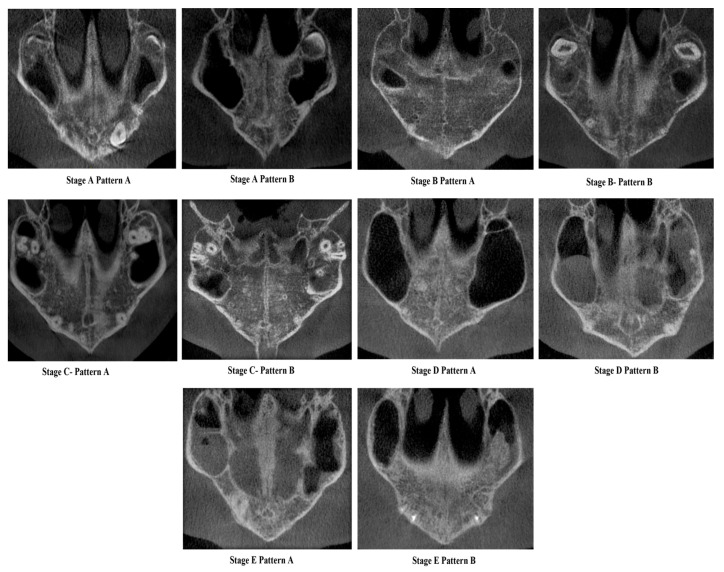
Cone Beam Computed Tomographic images of the New classification of Midpalatine Suture Staging with five stages and two patterns per stage.
